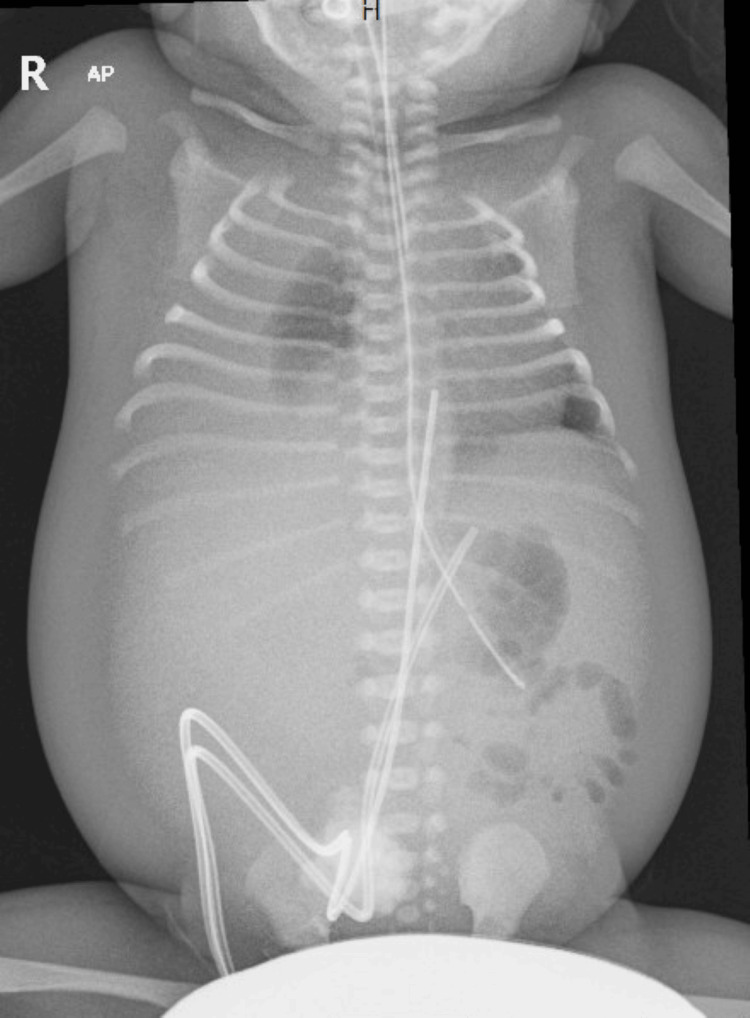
Postnatal chest and abdomen radiograph showing features of hydrops fetalis, including marked ascites.
